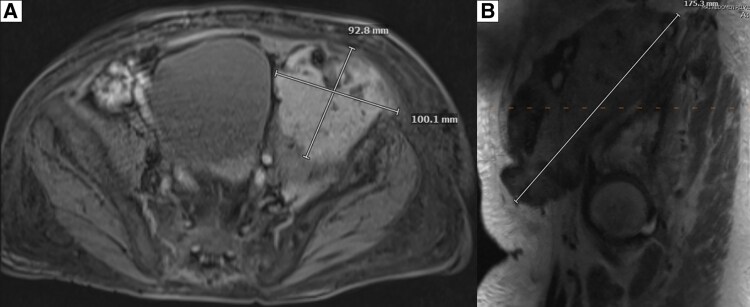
On the initial presentation, the magnetic resonance imaging (MRI) of the abdomen/pelvis shows a large left-sided pelvic mass measuring 10.1 cm × 9.3 cm transaxial (A) and 17.6 cm craniocaudal (B); dimensions are per formal radiology report.
